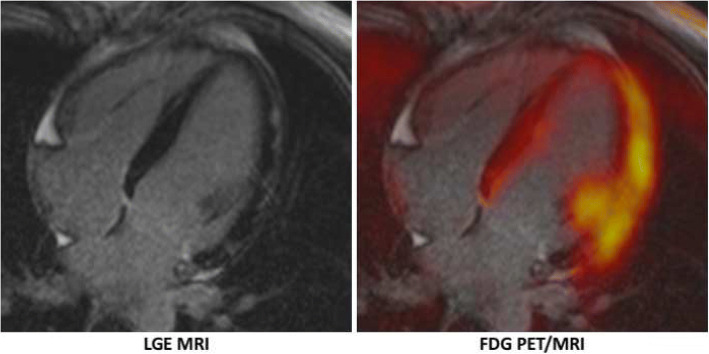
FDG PET/MRI in a case of myocarditis. Subepicardial, mostly band-shaped late gadolinium enhancement (LGE) on MRI in the lateral wall of the left ventricle. The FDG uptake on PET clearly exceeds LGE MRI indicating active infection exceeding the scarred areas
